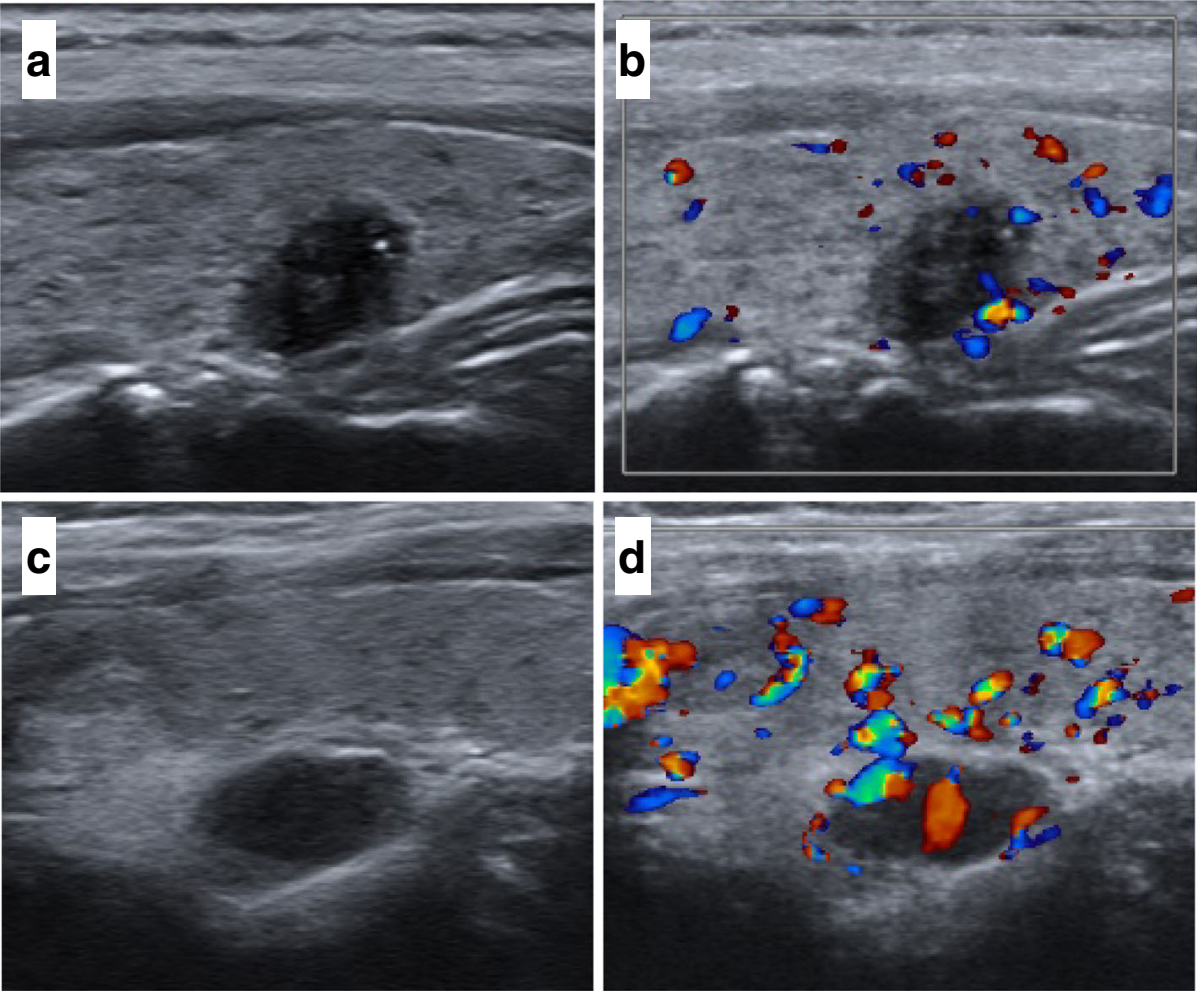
Cervical ultrasonography of a 50-year-old female patient. a and b showed a hypoechoic thyroid nodule measuring 11 mm× 7 mm near the inferior pole of the left thyroid lobe with irregular form, unclear border, micro-calcification. Little blood signal was detected in CDFI mode. c and d showed a hypoechoic right superior parathyroid lesion. Rich color blood flow signal was detected. The pathological results were papillary thyroid micro-carcinoma and parathyroid adenoma respectively
